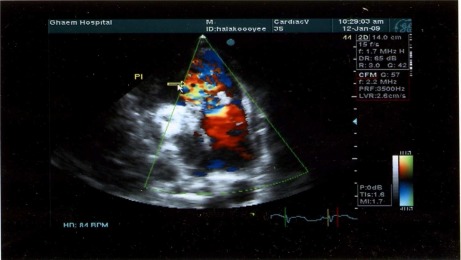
Parasternal short axis color Doppler imaging revealed severe free pulmonic insufficiency
